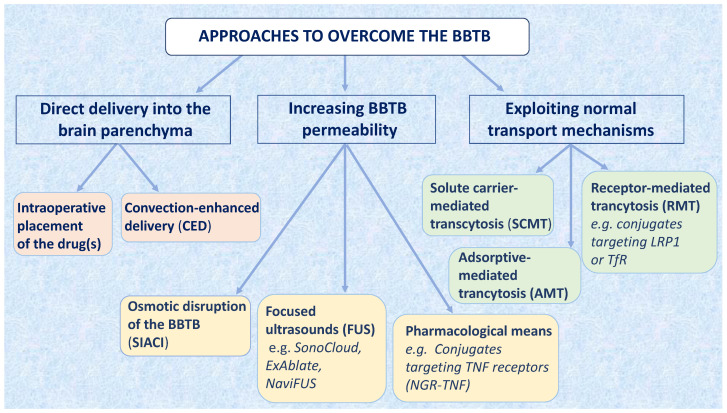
Approaches to overcome the BBTB. Three main classes of approaches to overcome the BBTB are shown. Each class is divided in different subclasses. Abbreviations: BBTB, blood–brain tumor barrier; FUS, focused ultrasound; SCMT, solute carrier-mediated transcytosis; SIACI, superselective intraarterial cerebral infusion; Tfr, transferrin receptor; TNFR, tumor necrosis factor receptor.
